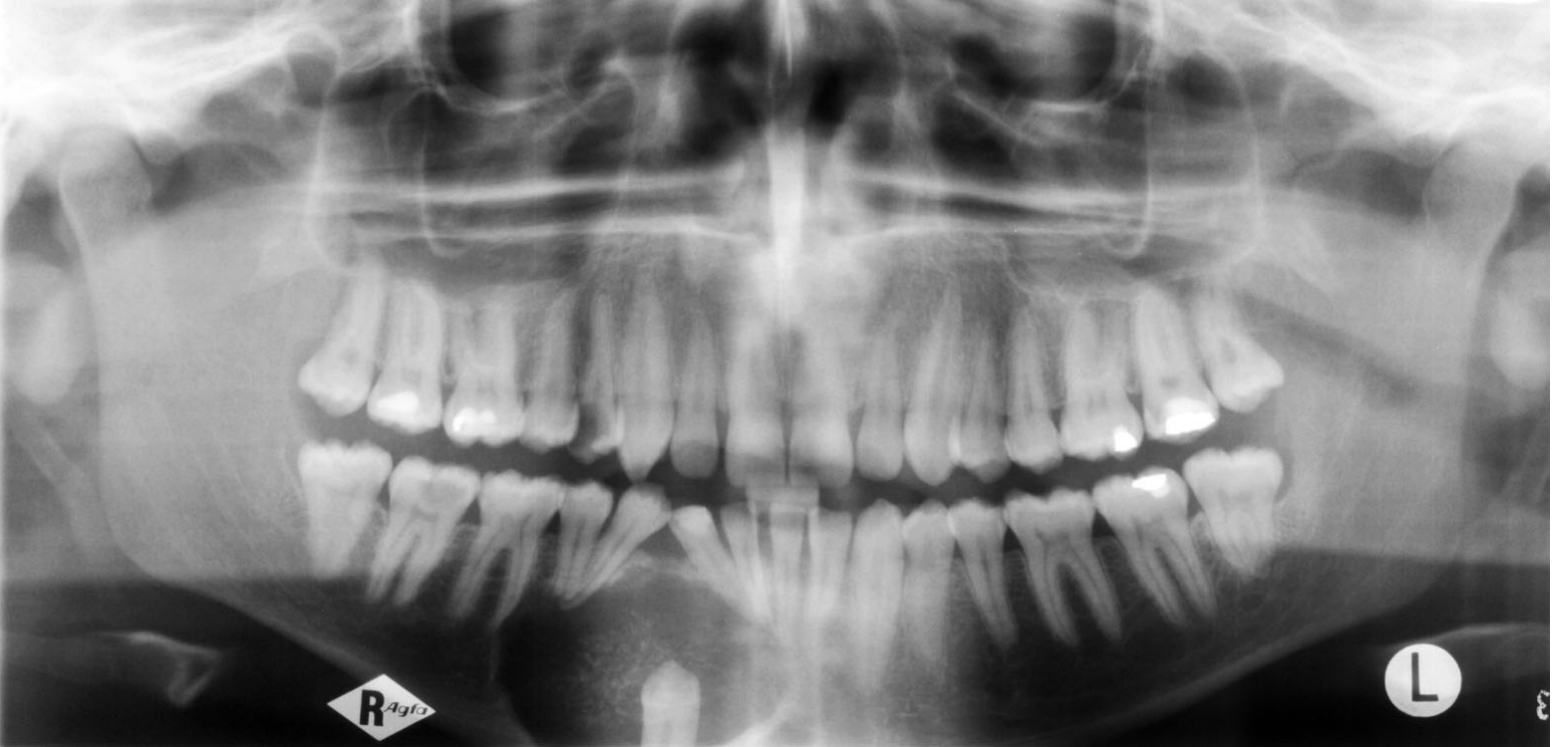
Panoramic radiograph before therapy. Unicystic radiolucent lesion in the lawer right jaw with a comparatively clear demarcation. The tooth 43 is located on the floor of this process. There are no resorption of the root apices.
